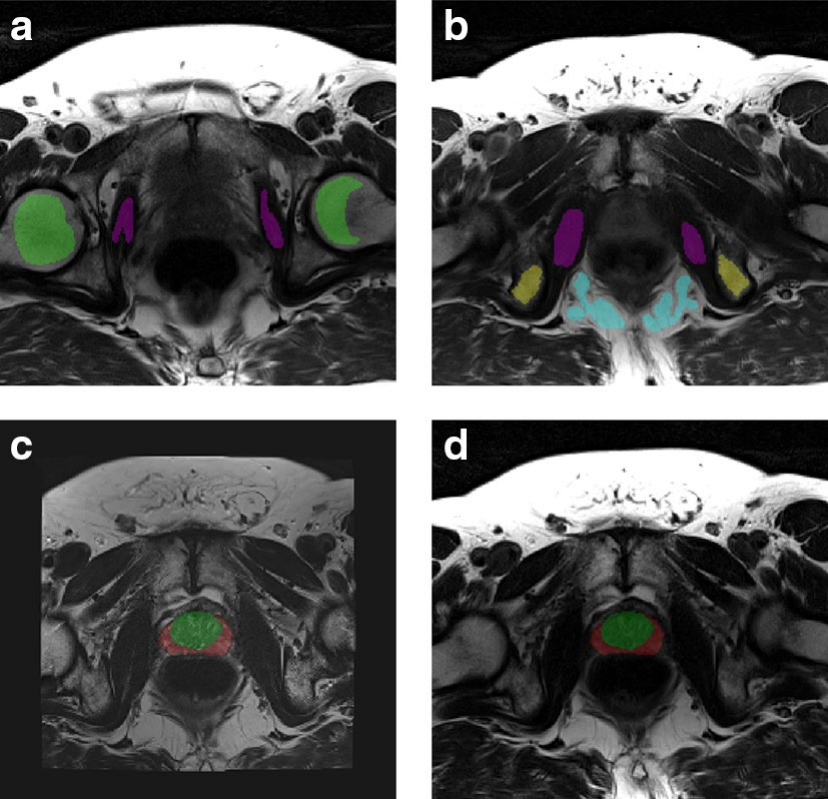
a, b Two slices of the transversal multi-echo spin echo (MESE) image (TE = 106 ms) of an asymptomatic volunteer, with manual delineations within the reference regions. Purple indicates the obturator internus muscle, yellow the ischial tuberosity (pelvic bone), blue the ischioanal fossa (fat) and green the yellow bone marrow in the femoral heads. c Transversal T2-weighted image registered to the MESE image space, with co-registered prostate segmentation. The peripheral zone is red, while the remaining zones (transitional zone, central zone and anterior fibromuscular stroma) are green. d Transversal MESE image (TE = 106 ms) with registered manual prostate segmentations
